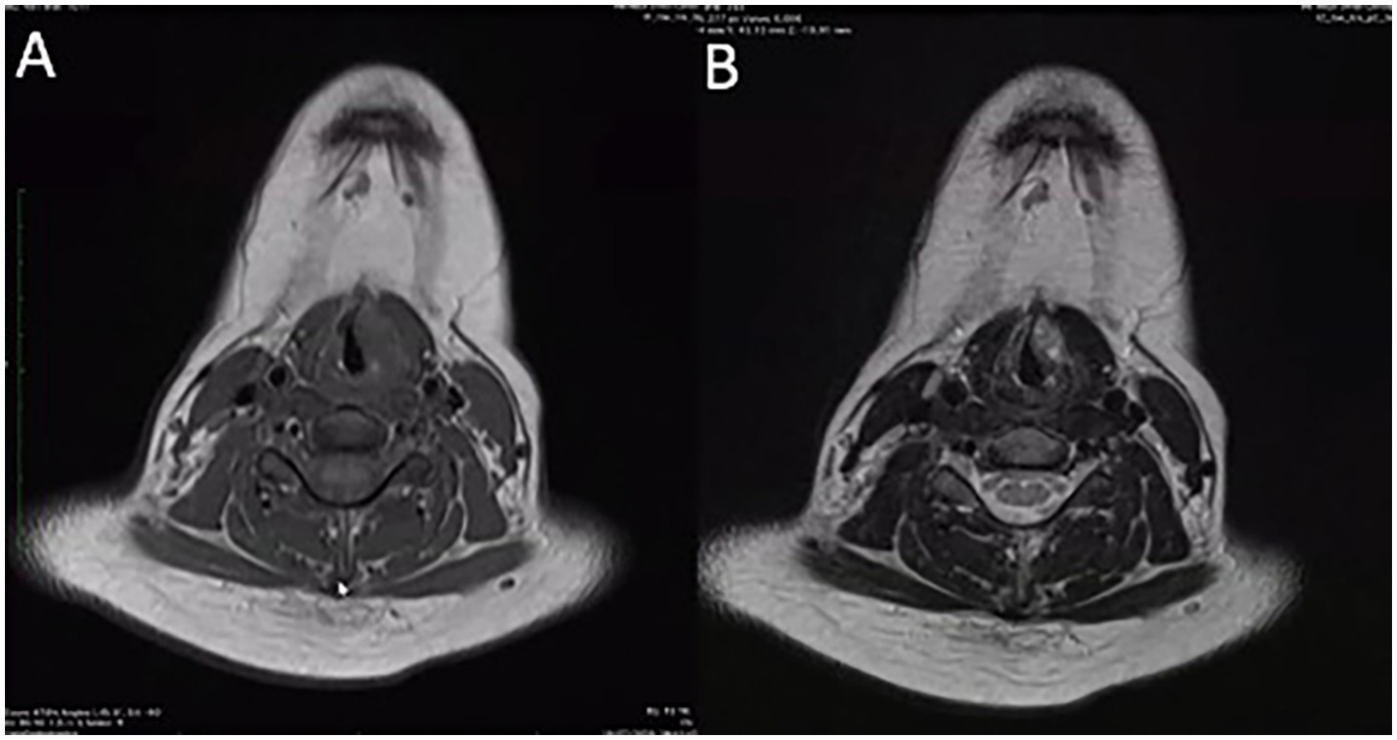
Contrast magnetic resonance imaging of the head and neck. (A) A heterogenous lesion located at the anterior 2/3rd of left paraglottic space measuring 1.9 cm × 0.8 cm × 1.5 cm, that was low signal on T1, (B) heterogenous hyperintense on T2, and enhanced on post-contrast. The lesion abuts the left thyroid lamina without any radiological evidence of erosion.
